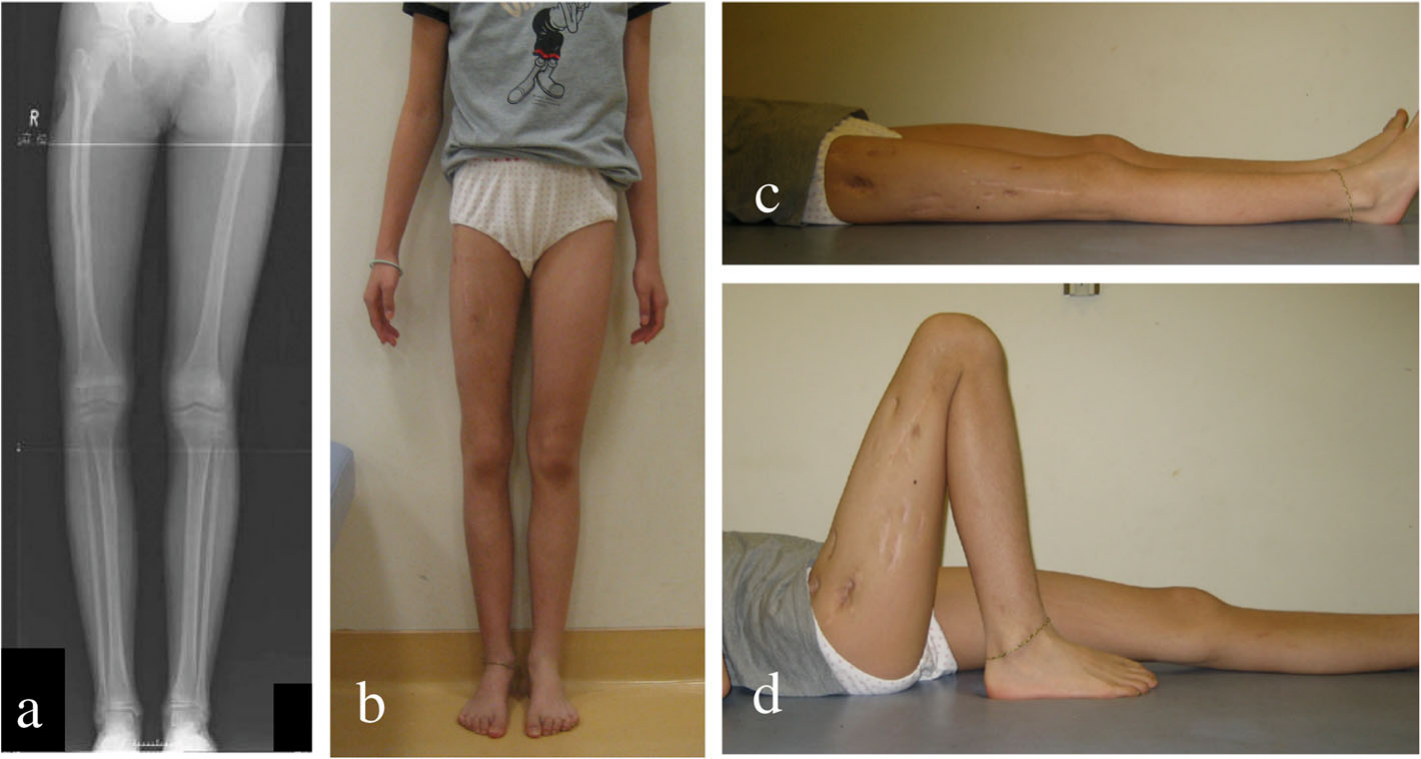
At the age of 12 years, 10 months. a Frontal radiograph of both limbs. b External appearance of both limbs. c External appearance of knee extension. d External appearance of knee flexion. Alignment of the right femur was successful and most of the difference in length was corrected
